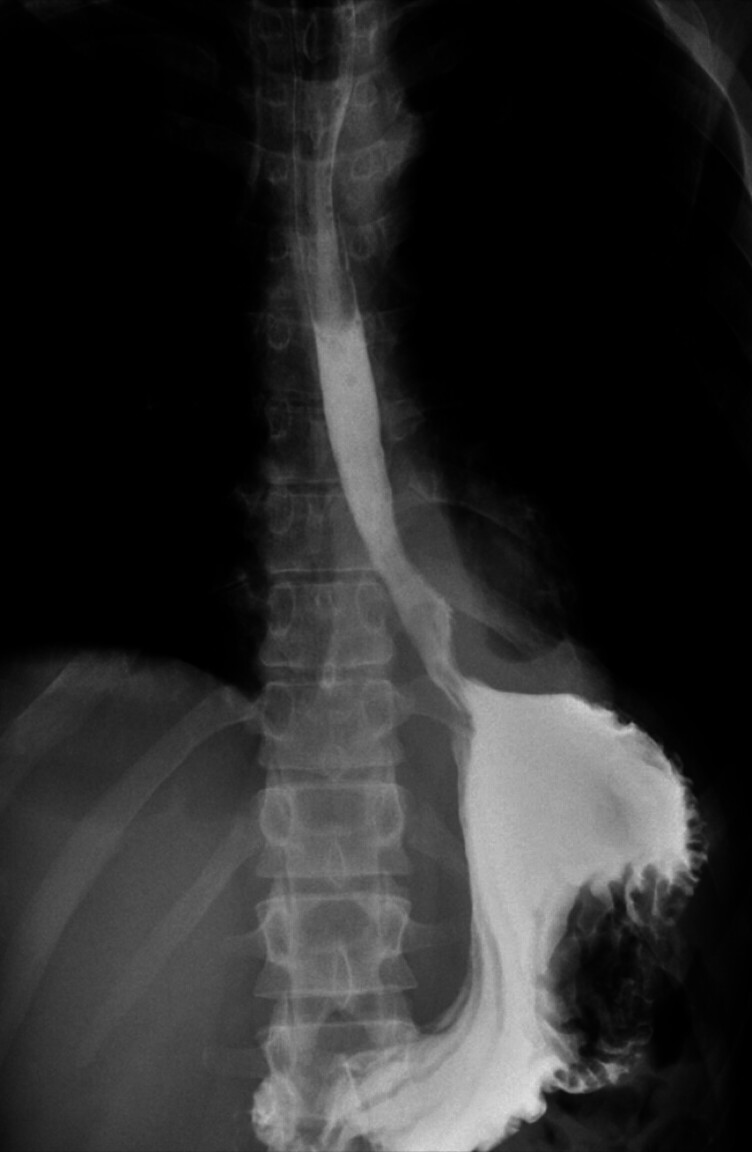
X-ray with oral contrast agent.
